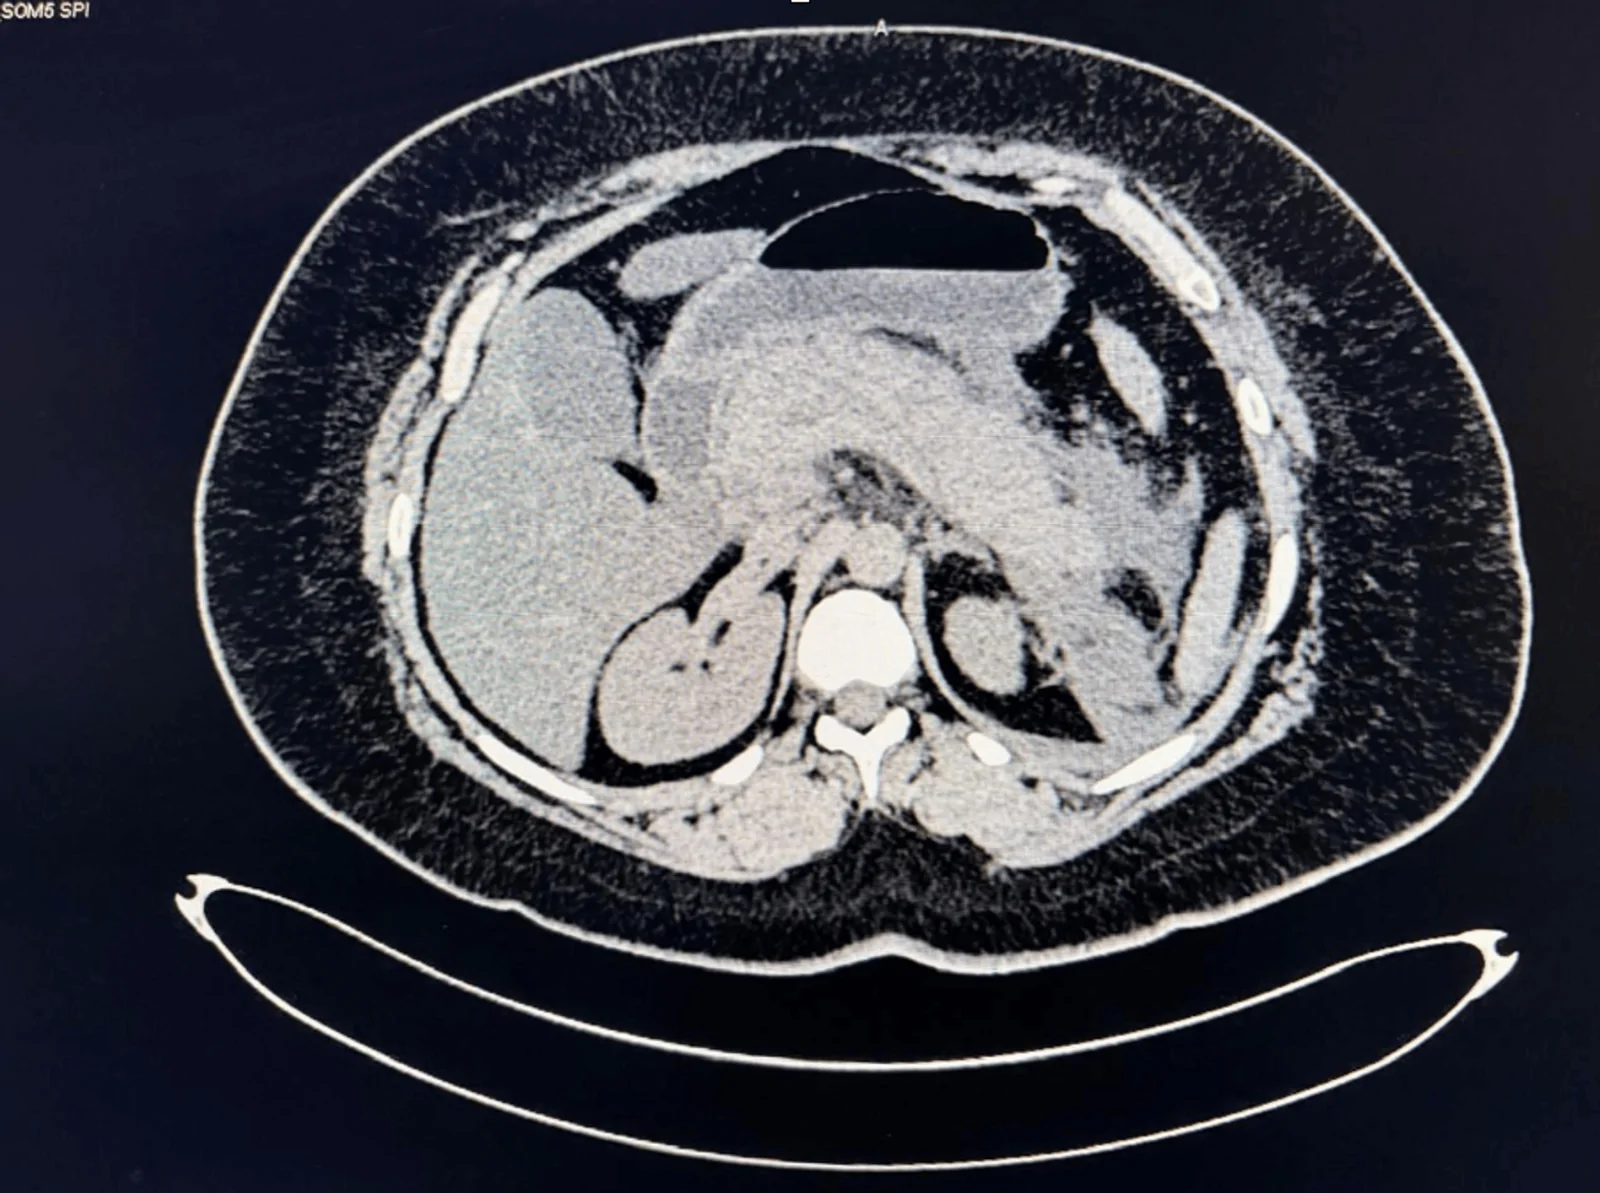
Non-contrast abdominal CT scan performed at presentation. Axial CT image demonstrating significant pancreatic inflammation and peripancreatic fat stranding, consistent with Balthazar grade C pancreatitis.
